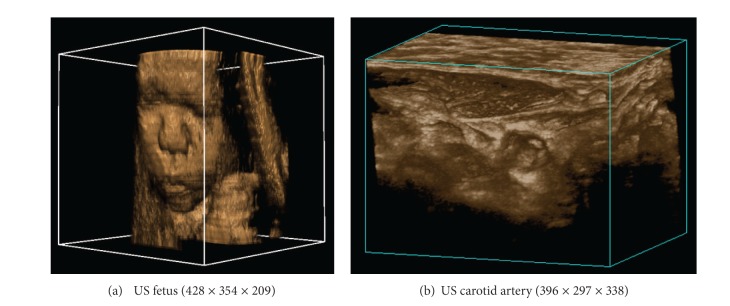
Real ultrasound volumes for experiment.
